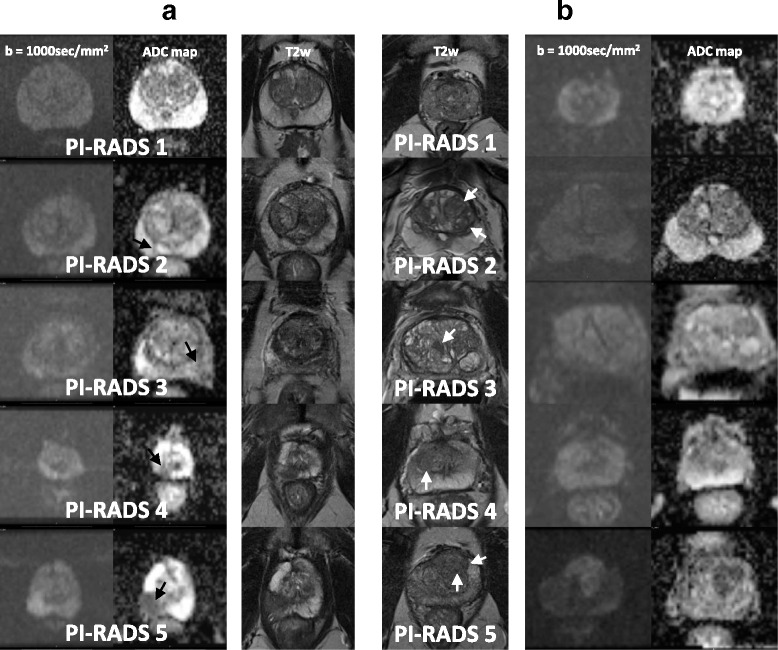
PI-RADS™ v2 assessment categories: Fig. 1a depicts typical findings in the peripheral zone and Fig. 1b depicts typical findings in the transition zone. a. Peripheral zone shows PI-RADS assessment categories with DWI as dominant sequence. 1. No abnormality (i.e. normal) on the high b-value DW image and on the corresponding ADC map as well as on the T2w image. 2. Isointense signal of the peripheral zone on the high b-value DW image with an indistinct linear hypointense lesion on the ADC map (arrow) with corresponding T2w hypointense signal. 3. Isointense/mildly hyperintense signal of the peripheral zone on the high b-value DW image and with a focal mildly/moderately hypointense indistinct lesion on the ADC map (arrow). T2w image shows heterogeneous signal intensity of the peripheral zone. 4. Focal markedly hyperintense lesion on high b-value DW image with corresponding markedly hypointense signal intensity on the ADC map (arrow). Lesion size is < 1.5 cm on axial images. T2w image shows a circumscribed homogenous hypointense lesion. 5. Same as 4, but lesion size is ≥ 1.5 cm in greatest dimension (arrow). Definite extraprostatic extension/invasive behaviour (not shown) would also qualify for this category. b Transition zone shows PI-RADS assessment categories with T2w as dominant sequence: 1. Homogeneous intermediate signal intensity (normal) on T2w image and no abnormality on high b-value DW image and ADC map. 2. Circumscribed (arrows) hypointense or heterogeneous encapsulated nodule(s) (BPH). High b-value DW image shows normal signal intensity and indistinct hypointense signal on ADC map. 3. Lesion with heterogeneous signal intensity with obscured margins (arrow). High b-value DW image of the lesion is mildly hyperintense and moderately hypointense on the ADC map. This category includes lesions that do not qualify as 2, 4, or 5. 4. Lenticular (arrow) or non- circumscribed, homogeneous, moderately T2w hypointense lesion, < 1.5 cm in greatest dimension. High b-value DW image of the lesion shows markedly hyperintense signal and markedly hypointense signal on the ADC map. 5. Same as 4, but lesion size is ≥ 1.5 cm in greatest dimension (arrows). Definite extraprostatic extension/invasive behaviour (not shown) would also qualify for this category
